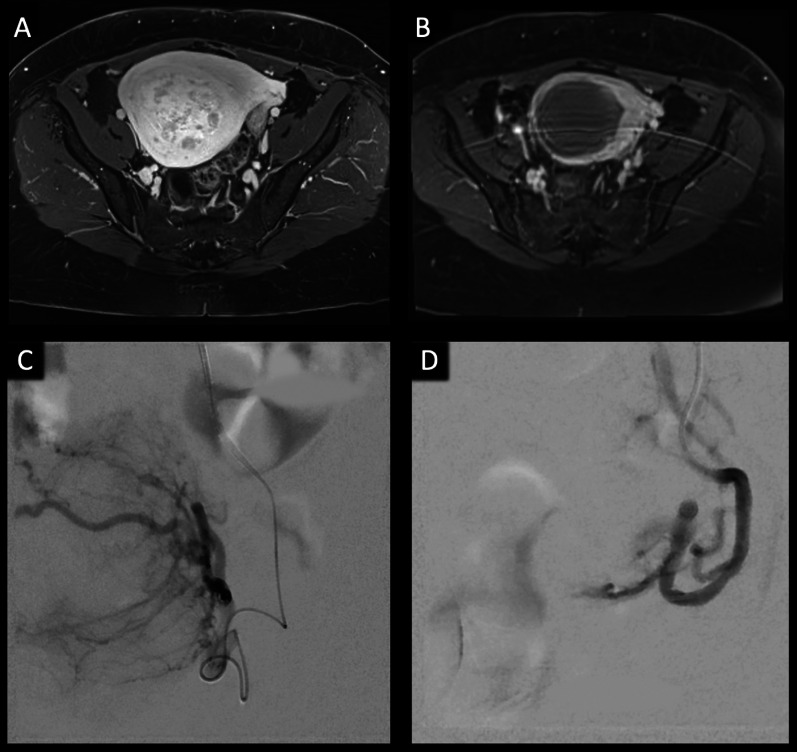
UAE. T1-weighted contrast-enhanced MRI of the pelvis before (A) and after (B) UAE in a patient showing a good response to treatment. The hypointense areas in the center of the tumor mass indicate tumor necrosis. Digital subtraction angiography of the uterine artery before (C) and after (D) embolization showing stasis in the uterine artery
